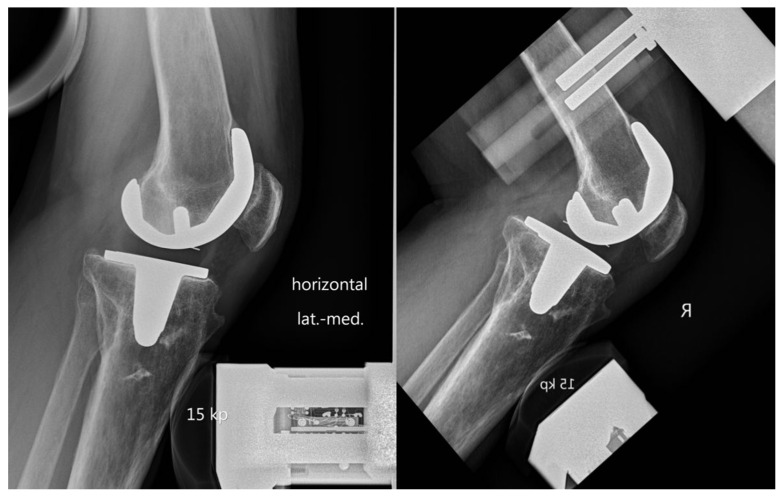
Stress radiographs showed increased posterior translation in posterior drawer position in 90° flexion compared to 30° flexion.
